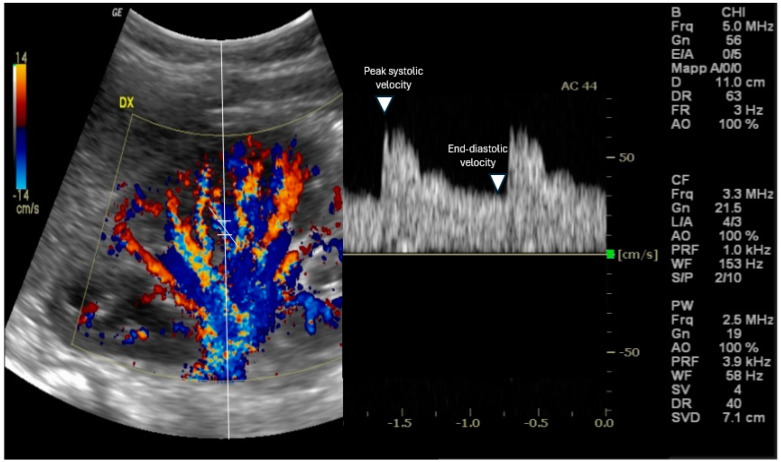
Evaluation of the renal resistive index using Doppler ultrasound. The transducer is placed in an interlobar artery, and the spectral Doppler examines the peak systolic and end-diastolic velocities.
